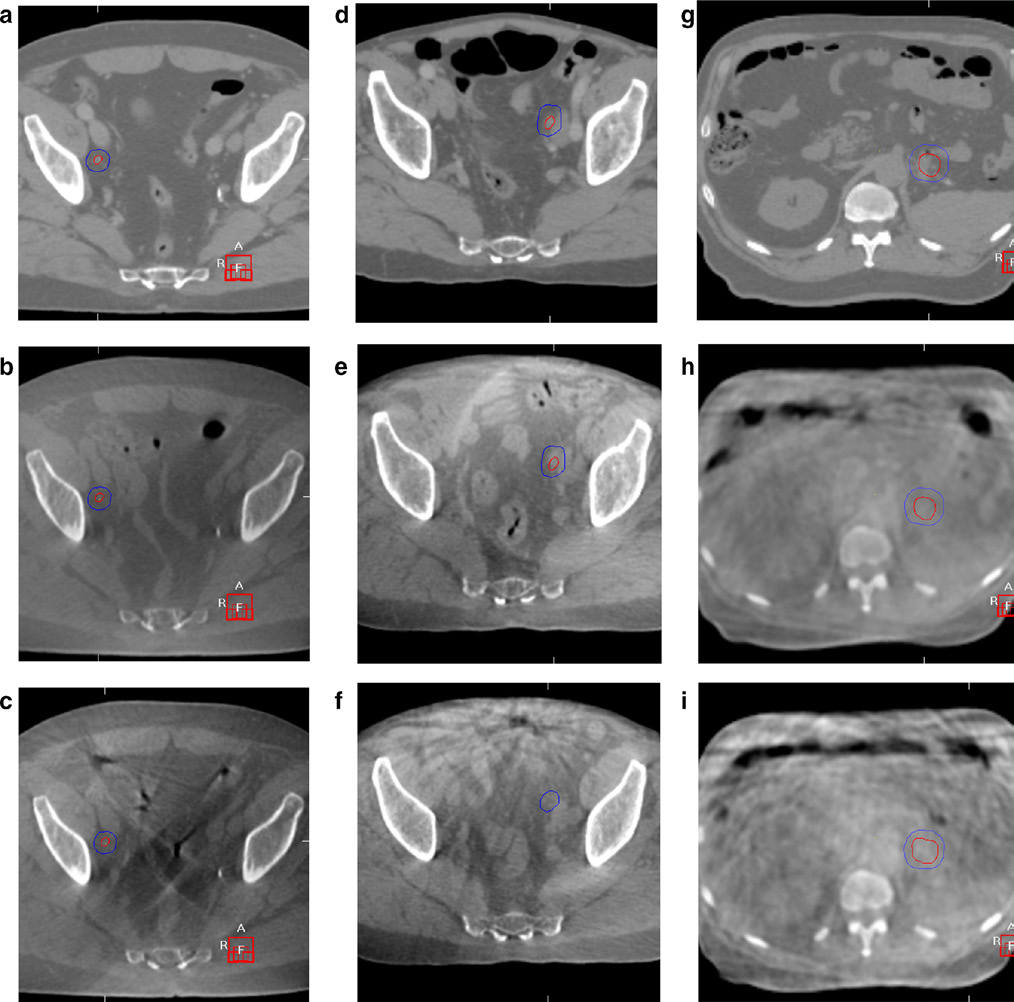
Planning CT and CBCT images with/without IM and i.v. buscopan for three patients. GTV and PTV are shown in each image. CBCT, cone beam CT; GTV, gross tumour volume; PTV, planning target volume.
